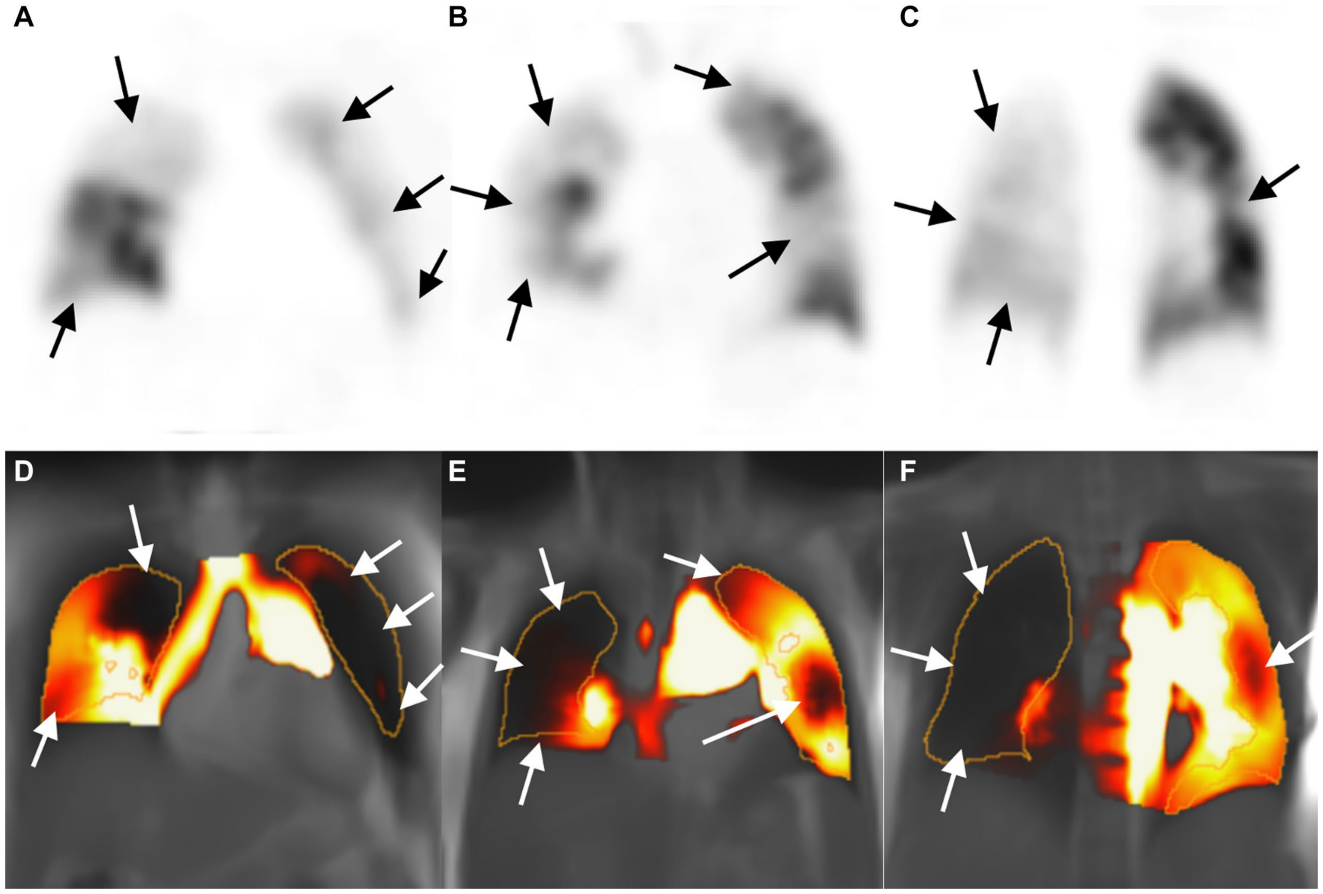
Images of a 53-year-old woman with chronic thromboembolic pulmonary hypertension. Perfusion defect in SPECT perfusion (A–C, arrow) and corresponding PW-PREFUL MRI (D–F, arrow) located in the whole right lung (except for subsegment IV and segment V) and in the left segments III, IV, and V, and subsegment VI. MRI, Magnetic resonance imaging; PREFUL, phase-resolved functional lung; PW, perfusion-weighted; SPECT, single-photon emission computed tomography.
